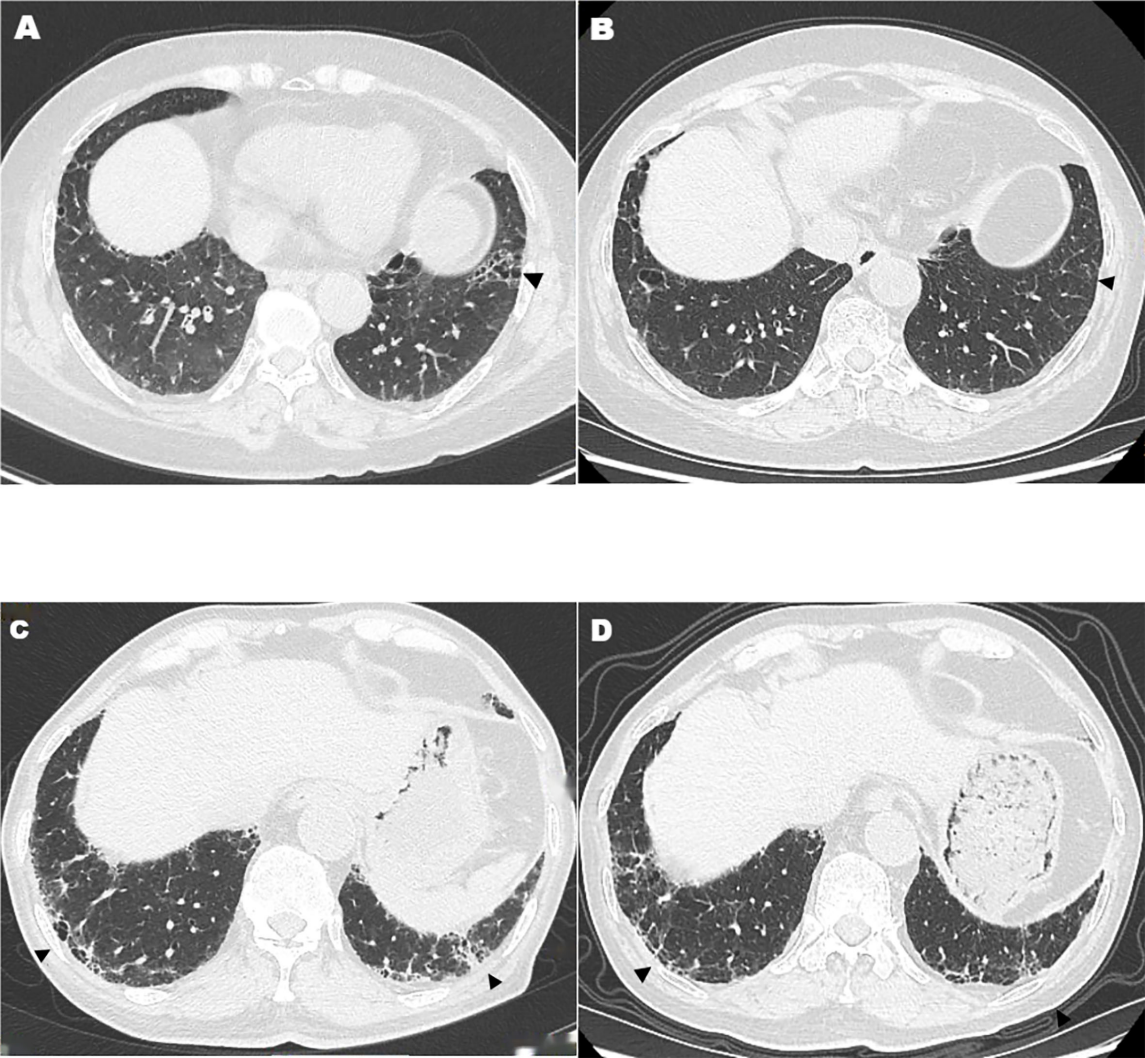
High-resolution computed tomography features after tofacitinib plus iguratimod treatment. Arrowheads show decreased fibrotic lesions that were partly absorbed, attenuated, or evolved into ground-glass opacity (A–D).
